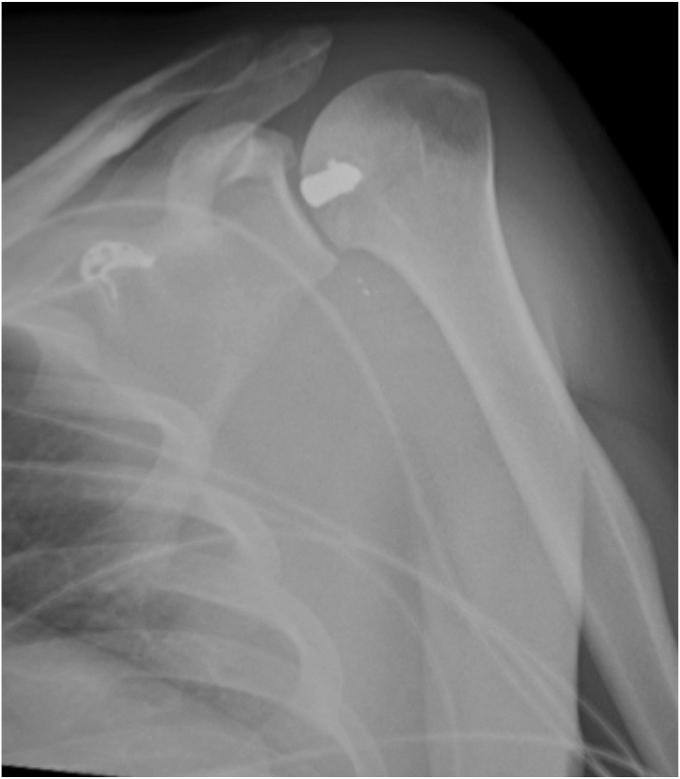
Initial injury radiograph, ballistic embedded in the humeral head.
